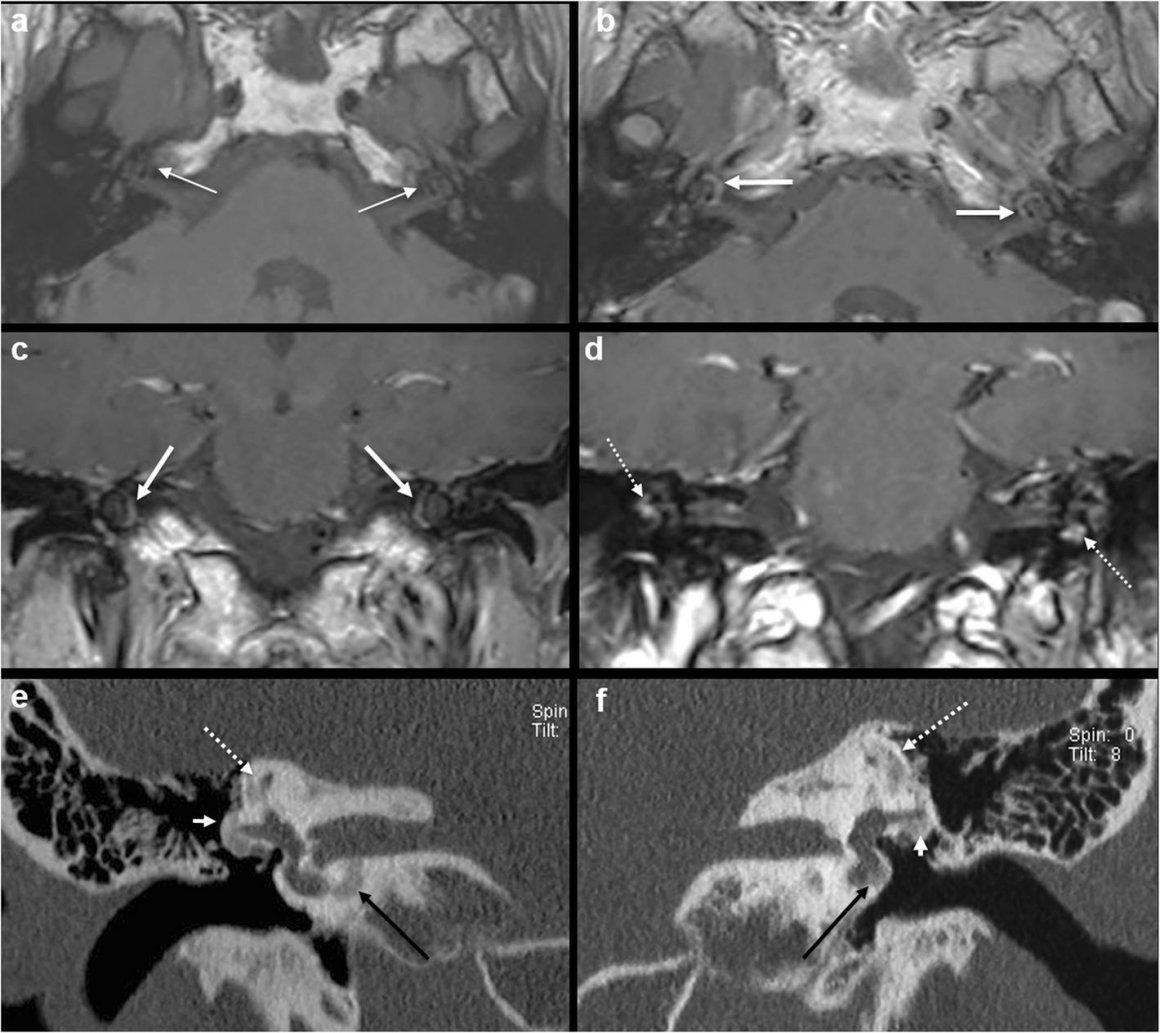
A 57-year-old female patient with left-sided SNHL (case 3). Axial T1W MR image (a) shows intermediate T1 signal in in bilateral pericochlear regions (arrows). Contrast-enhanced axial T1W MR image (b) and contrast-enhanced coronal T1W MR image (c) show ring-like enhancement in bilateral pericochlear regions (arrows). Contrast-enhanced coronal T1W MR image (d) also shows patchy enhancement surrounding the SCC bilaterally (dashed arrows). Subsequent coronal HRCT images (e, f) confirm otosclerotic plaques adjacent to bilateral lateral SCC (short white arrow), superior SCC (dashed arrow) and in bilateral pericochlear regions (long black arrows)
